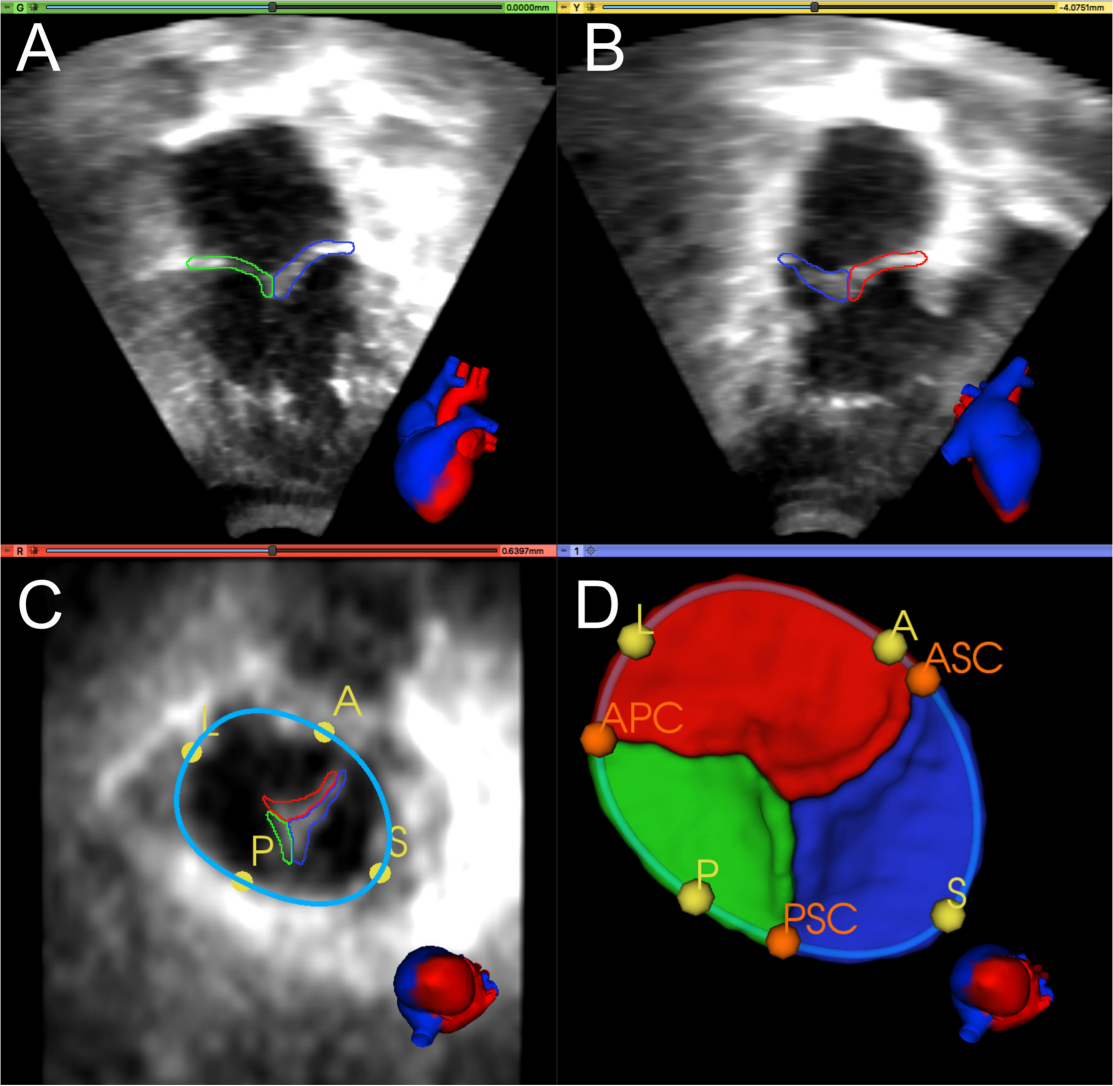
Manual segmentation and annotation of the tricuspid annulus and leaflets. (A) Apical 4-chamber view of 3D TTE MS frame showing the posterior (green) and septal (blue) leaflet; (B) Apical 2-chamber view of 3D TTE MS frame showing the anterior (red) and septal (blue) leaflet. (C) Ventricular view of 3D TTE MS frame with all three leaflets, the annular curve (light blue), and the quadrant landmarks (yellow): anterior (A), posterior (P), septal (S), and lateral (L). (D) 3D model of the segmented TV and the annular curve with APSL quadrant landmarks (yellow) and commissural landmarks (orange): anterior-septal commissure (ASC), posterior-septal commissure (PSC), and anterior-posterior commissure (APC). A heart avatar (red = left heart and aorta, blue = right heart, inferior and superior vena cava) is also provided for orientation of the echocardiographic views.
